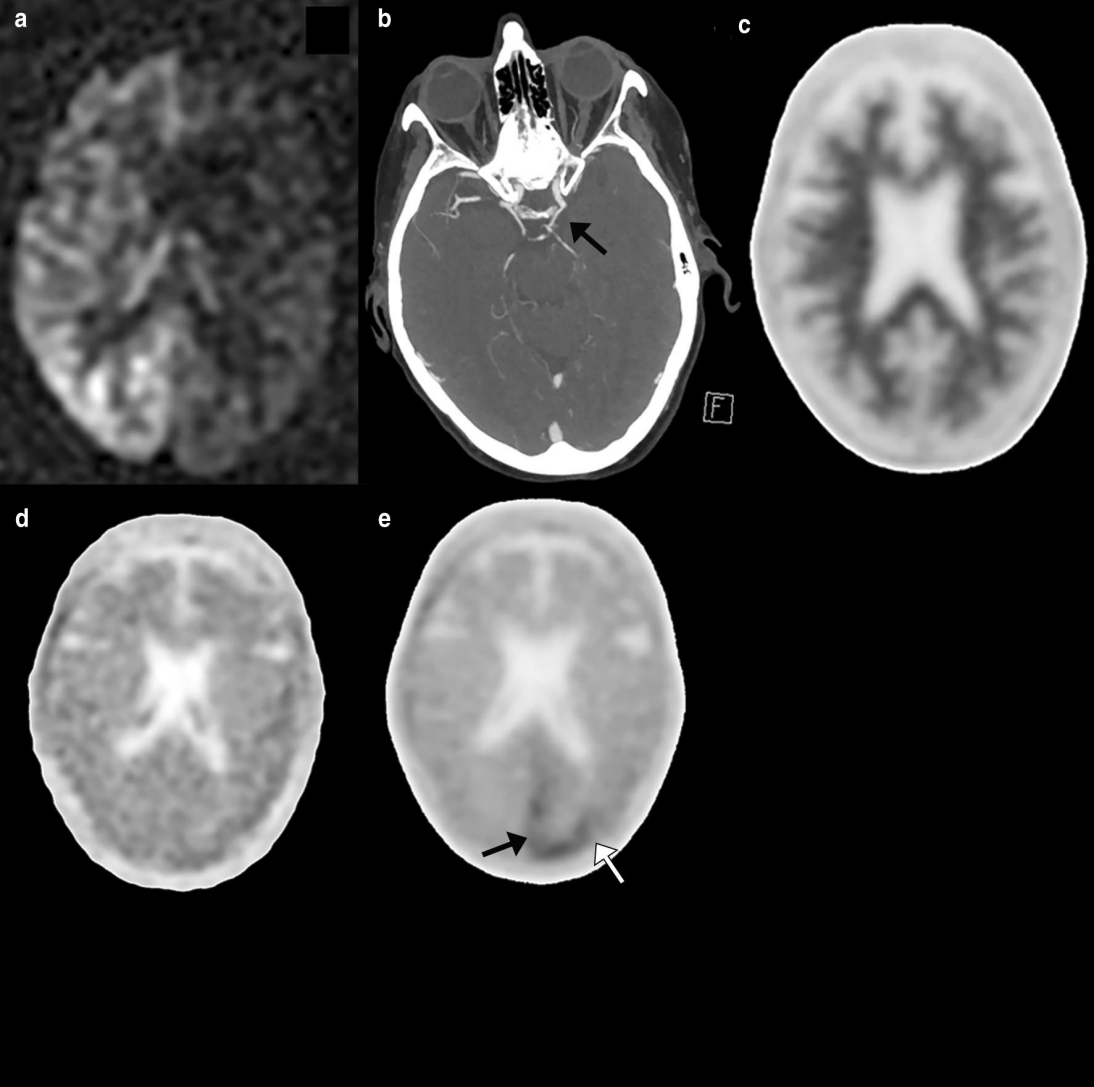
A 76-year-old female with symptomatic left carotid stenosis (70% by North American Symptomatic Carotid Endarterectomy Trial criteria) who presented with right homonymous hemianopia compatible with a left posterior cerebral artery stroke. Arterial spin labeling demonstrates hypoperfusion throughout the left cerebral hemisphere (a) and CTA demonstrates a fetal left posterior cerebral artery supplied by the left internal carotid artery (b). Florbetaben PET/CT demonstrates no to sparse amyloid plaque (c). Flortaucipir PET/CT prior to the event demonstrates no areas of cortical tau retention (d). Flortaucipir PET/CT following the event (e) demonstrates focal tau retention in the left posterior cerebral artery territory (arrows), likely as a response to cerebral ischemia
